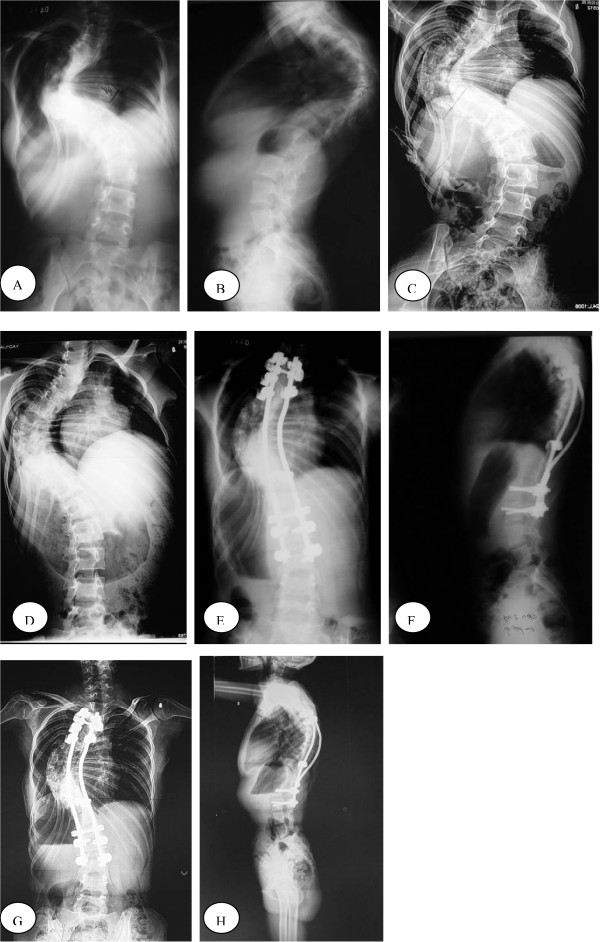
A 13-year-old girl with congenital scoliosis with defect of formation and segmentation. A-B: AP radiographs before treatment showed left thoracic scoliosis measured 98° and thoracic kyphosis measured 85°. C:Bending films showed right thoracic scoliosis only corrected to 90°. D:The left thoracic curve correction obtained with Halo-femoral traction was 24.1%. E-F:The major curve measured 50°after posterior spinal fusion with correction rate of 49.0%. The correction rate of kyphosis was 47.1%. G-H: AP and lateral radiographs at 18-month follow-up showed solid spinal fusion with a 53°left thoracic curve and a 45° thoracic kyphosis.
